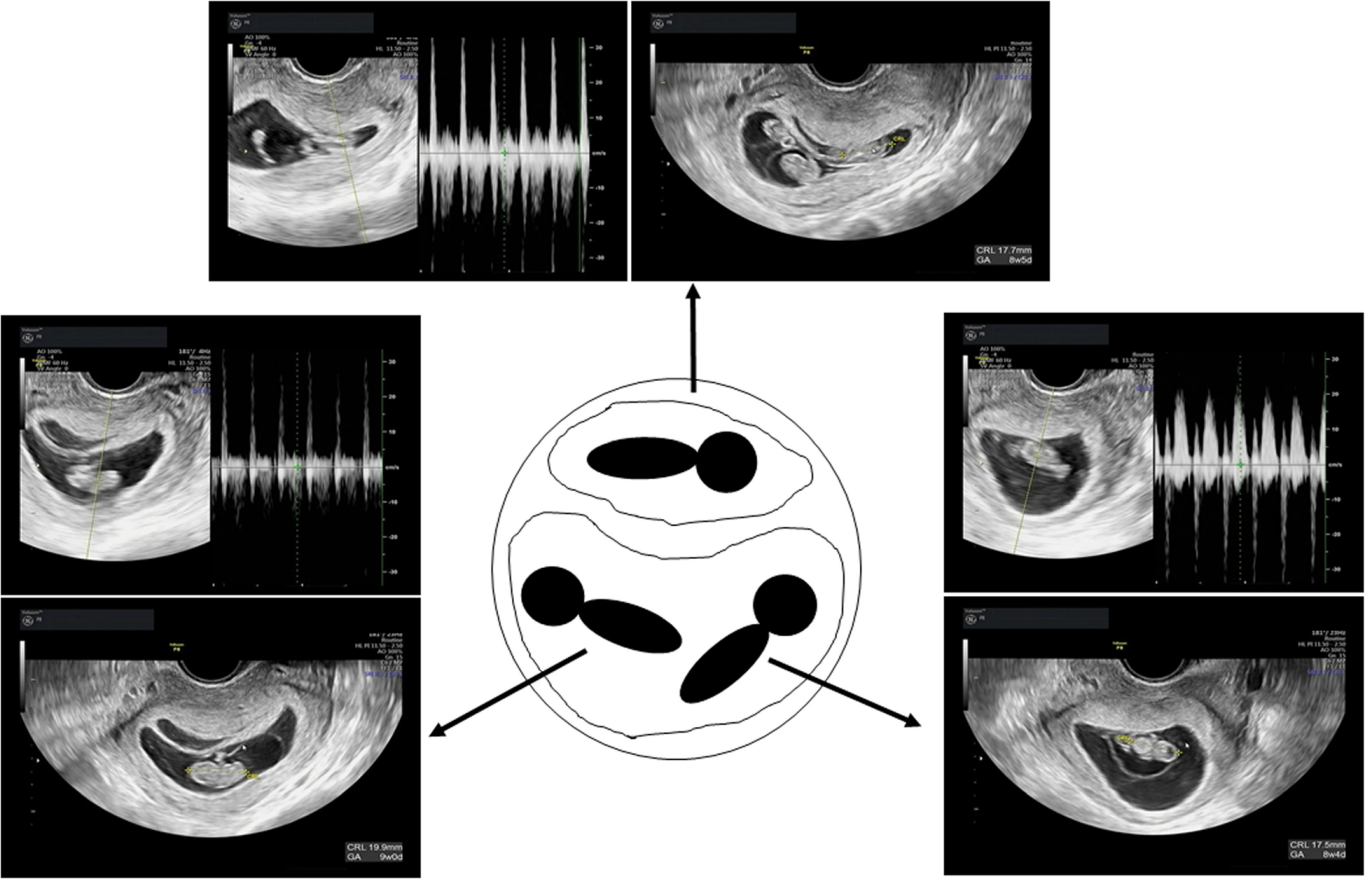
Representative images of transvaginal echocardiography at 8 weeks and 4 days. Three fetuses with heartbeats in the two gestational sacs were observed
